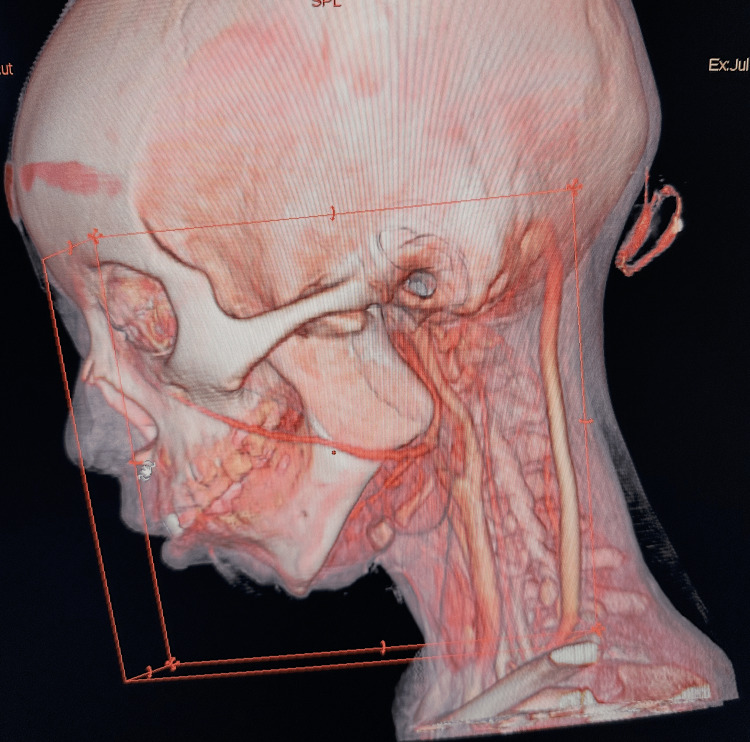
Preoperative computed tomography (CT) with three-dimensional reconstruction Preoperative three-dimensional CT reconstruction showing a marked left-sided hypoplasia of the condylar-ramus unit and a severe shortening of the mandibular ramus
